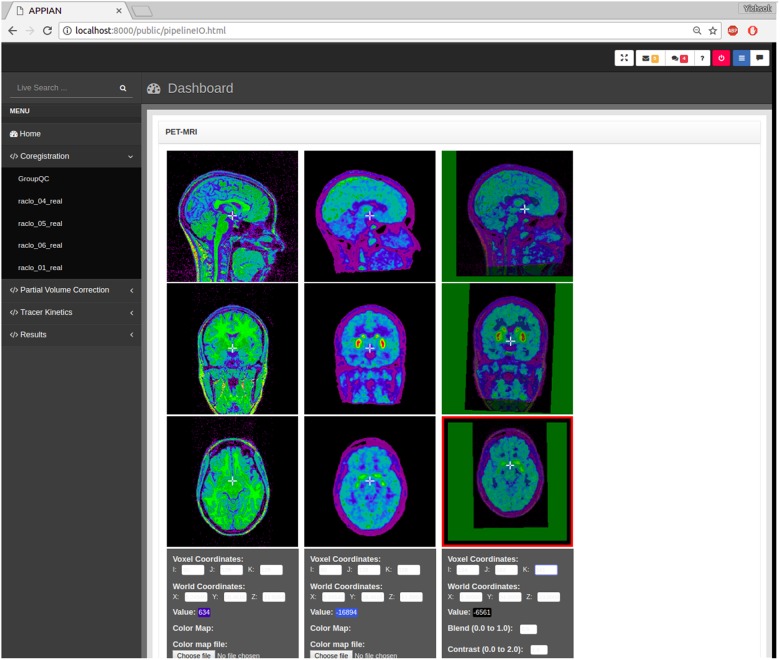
Output images produced by APPIAN can be viewed via a web browser-based dashboard. Visual QC for the coregistration stage can be performed by viewing the MRI, PET, and the fusion images of the two.
